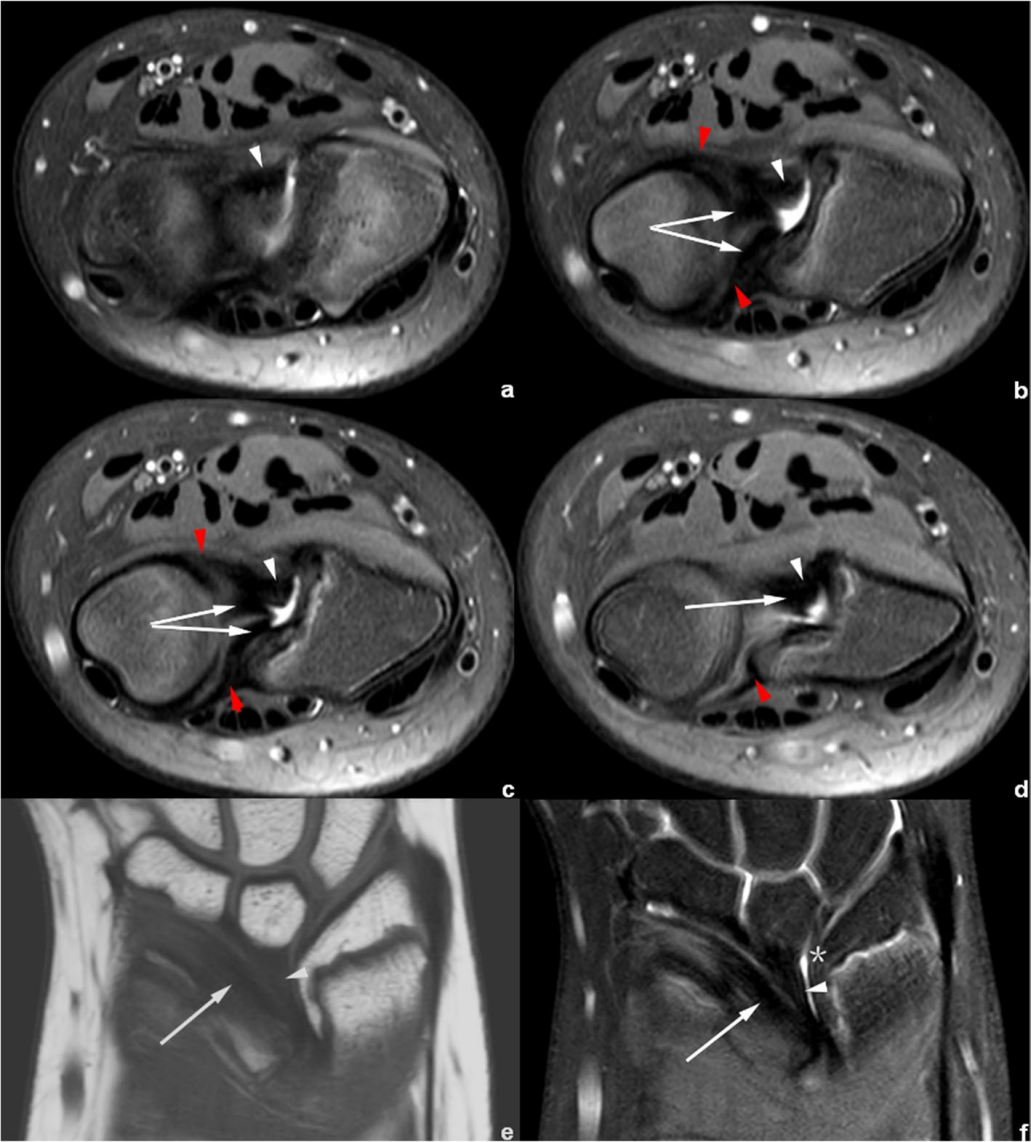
Right wrist MRI of the patient No. 1080. a–d Axial PDWI-FS images show the CD (white arrow), volar and distal radioulnar ligament (red arrowhead), and VL (white arrowhead). e Coronal T1WI image and f) coronal PDWI-FS image show the VL (white arrowhead) and a tilted, thickened CD (white arrow). Notice that the insertion of CD shifted proximally from the medial rim of distal radial articular surface (*)
